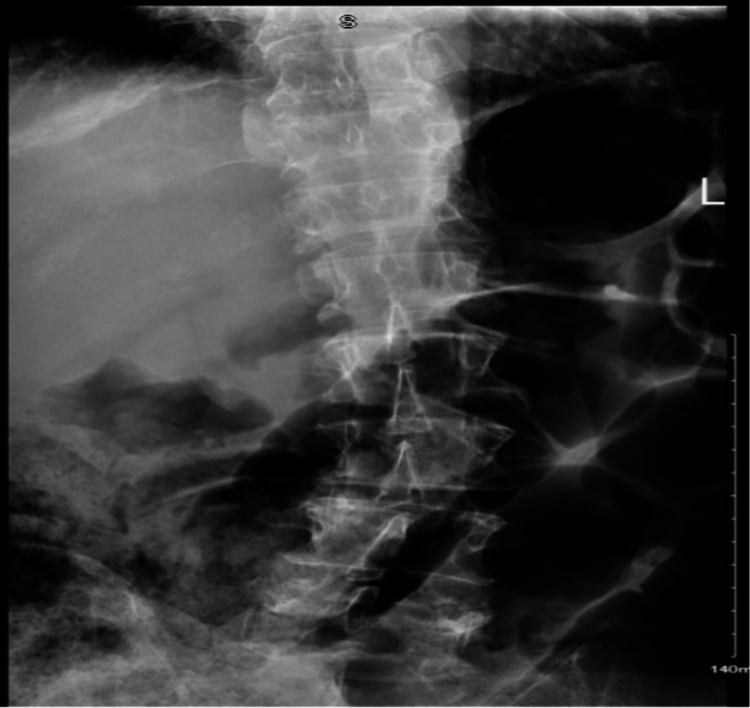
An x-ray of the lumbar spine was ordered and showed levoscoliosis with evidence of osteoarthritis and degenerative disc disease in L3-L4, L4-L5, and L5-1 vertebrae
